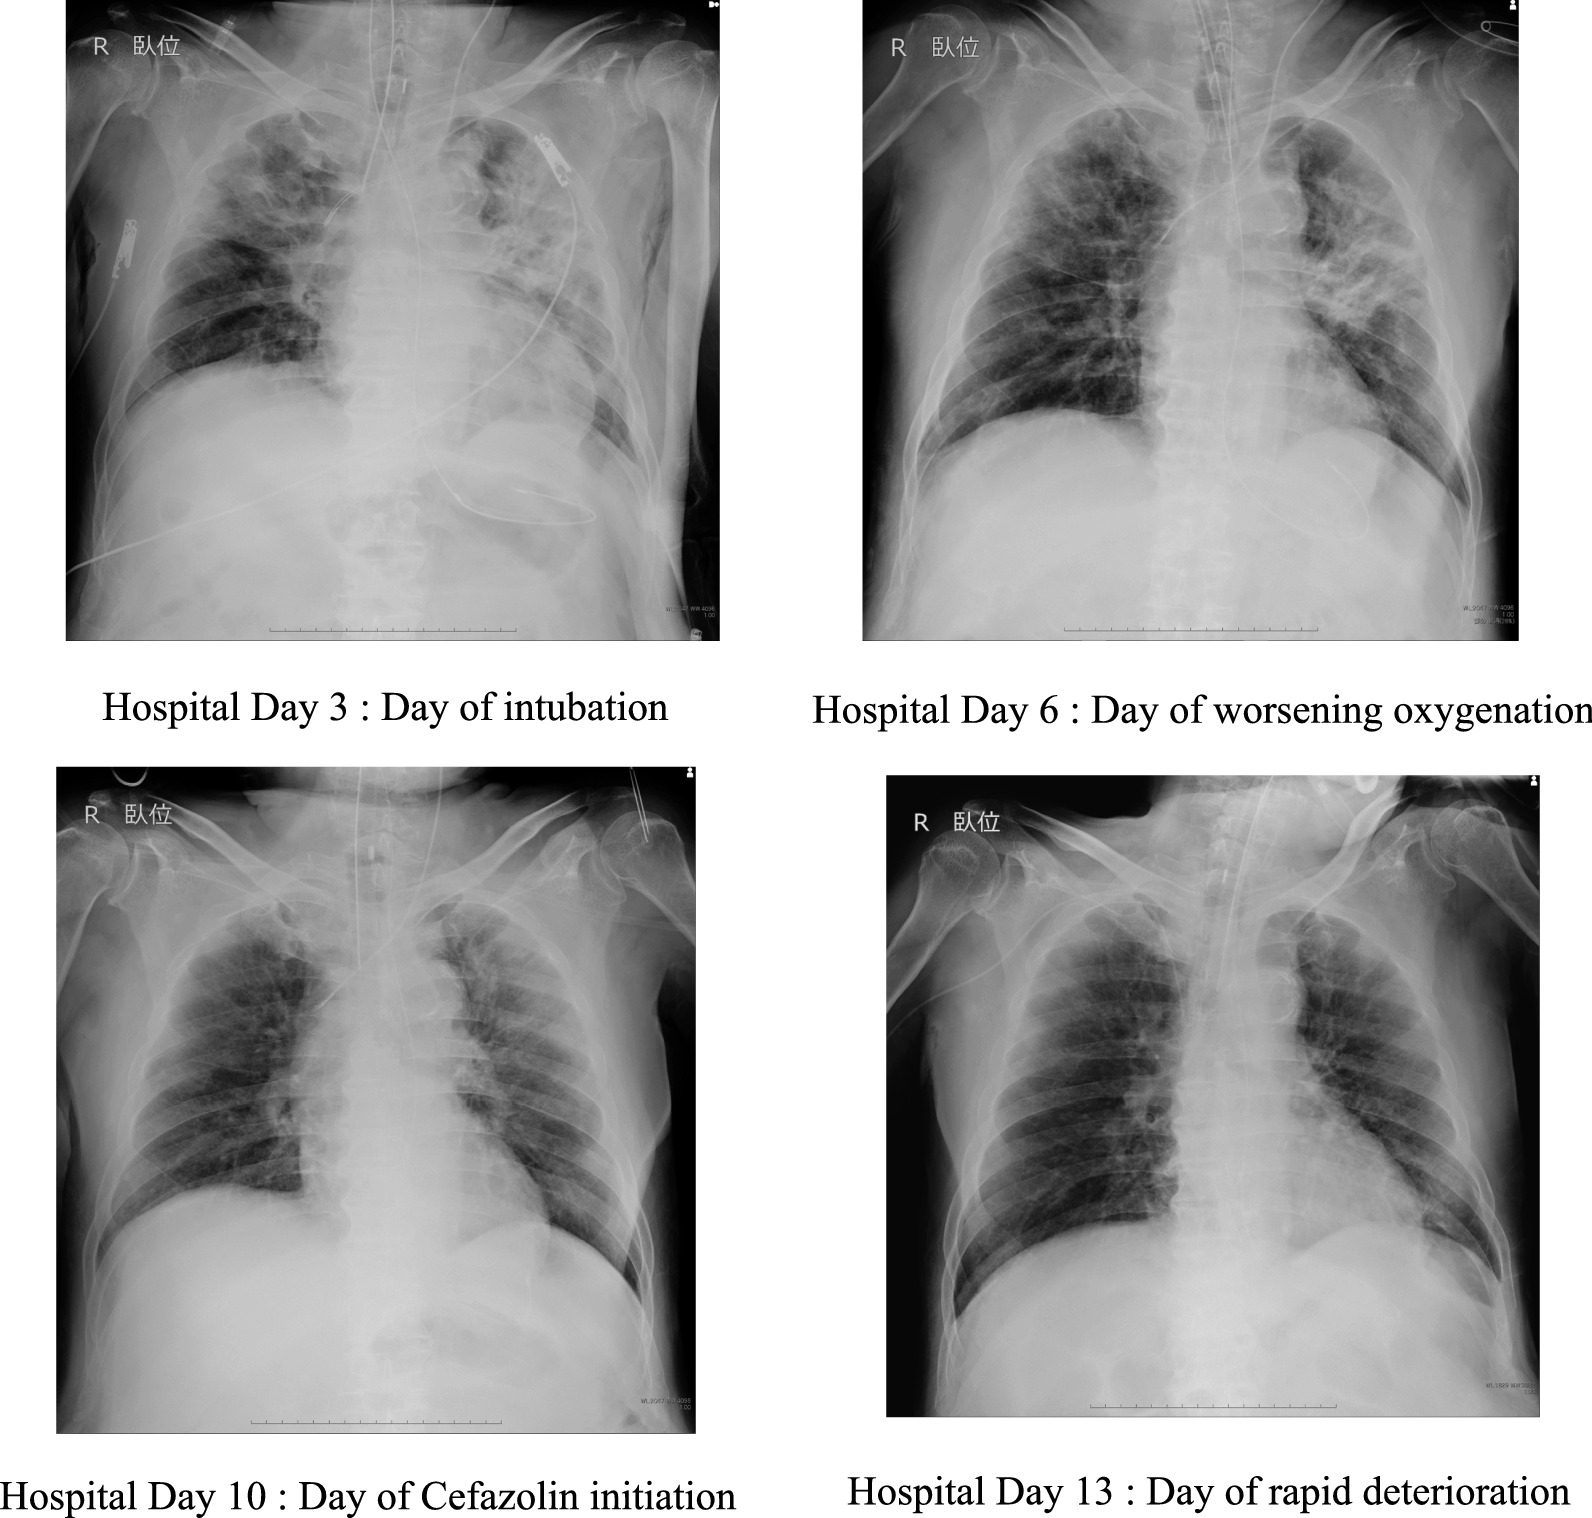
The serial chest radiographs of the patient
